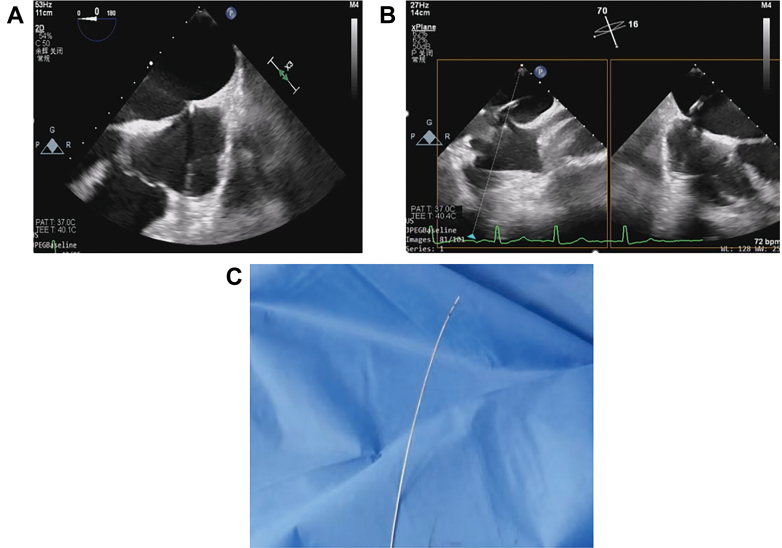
Transesophageal Echocardiography Images of Atrial Septal Puncture and Reverse-Shaped Puncture Needle (A) Measurement of atrial septal height at 0° in the reversed 4-chamber view. (B) Atrial septal puncture at 70° in the 2-chamber view. (C) Modified straightened configuration of the atrial septal puncture needle.
